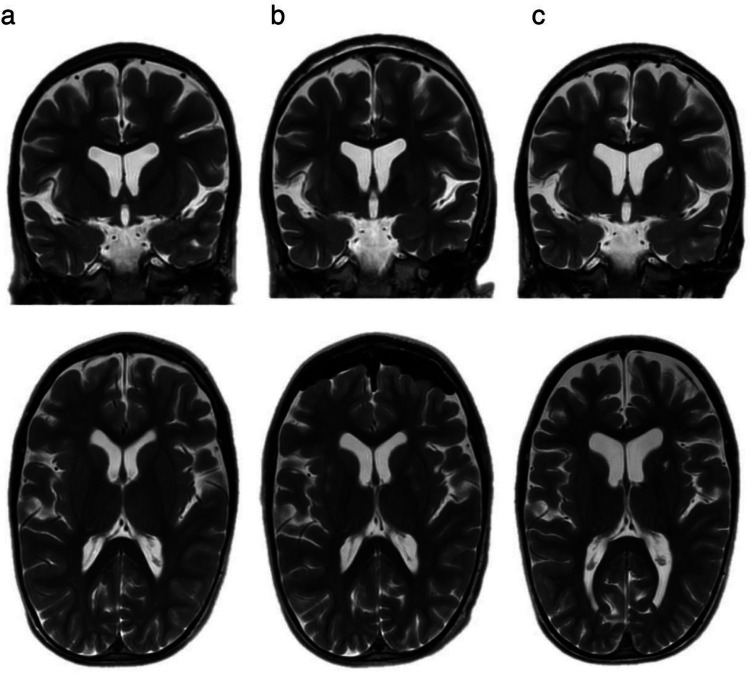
MRI scans of P2: A patient with cerebral atrophy. a Preoperative T2-weighted images in frontal and axial views. b Postoperative T2-weighted images in frontal and axial views showing post-puncture trajectories, with no signs of hemorrhagic stroke. c T2-weighted images on postoperative day 30 in frontal and axial views demonstrating a post-hemorrhagic infarction in the anterior-superior regions of the left putamen
